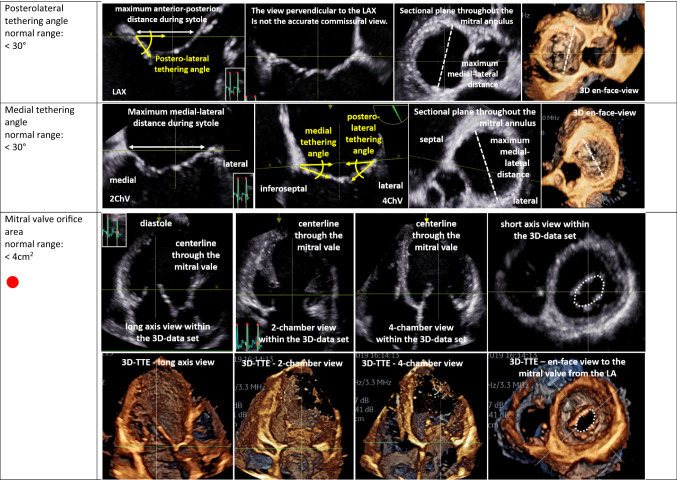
Echocardiographic parameters characterizing mitral valve (MV) deformation in SMR patients using conventional 2D echocardiography or 3D TTE
